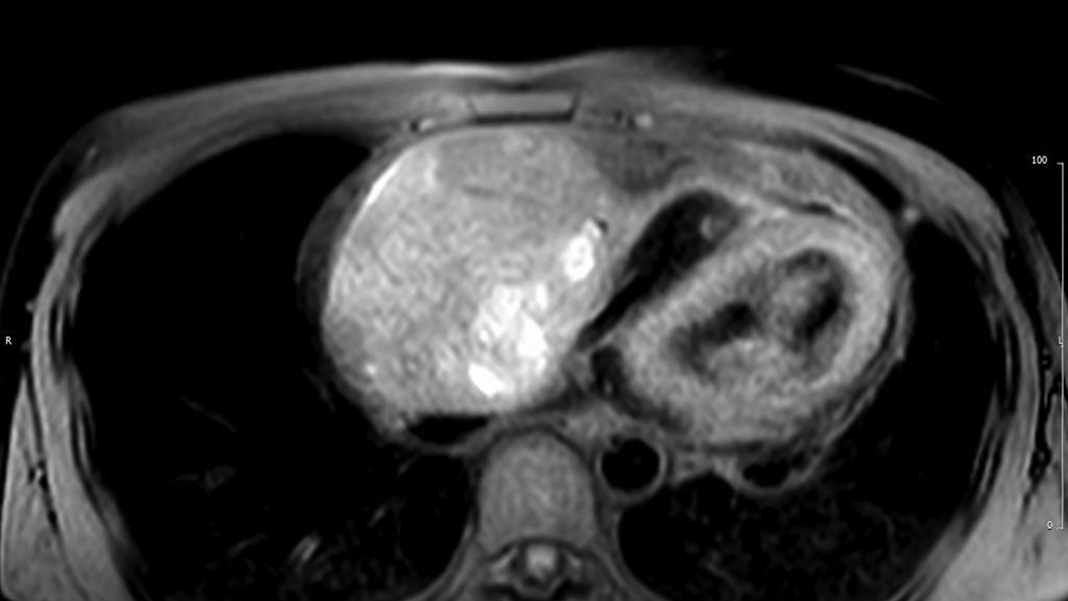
T_1_-Weighted Cardiac Magnetic Resonance Image Showing the Right Atrial Mass
